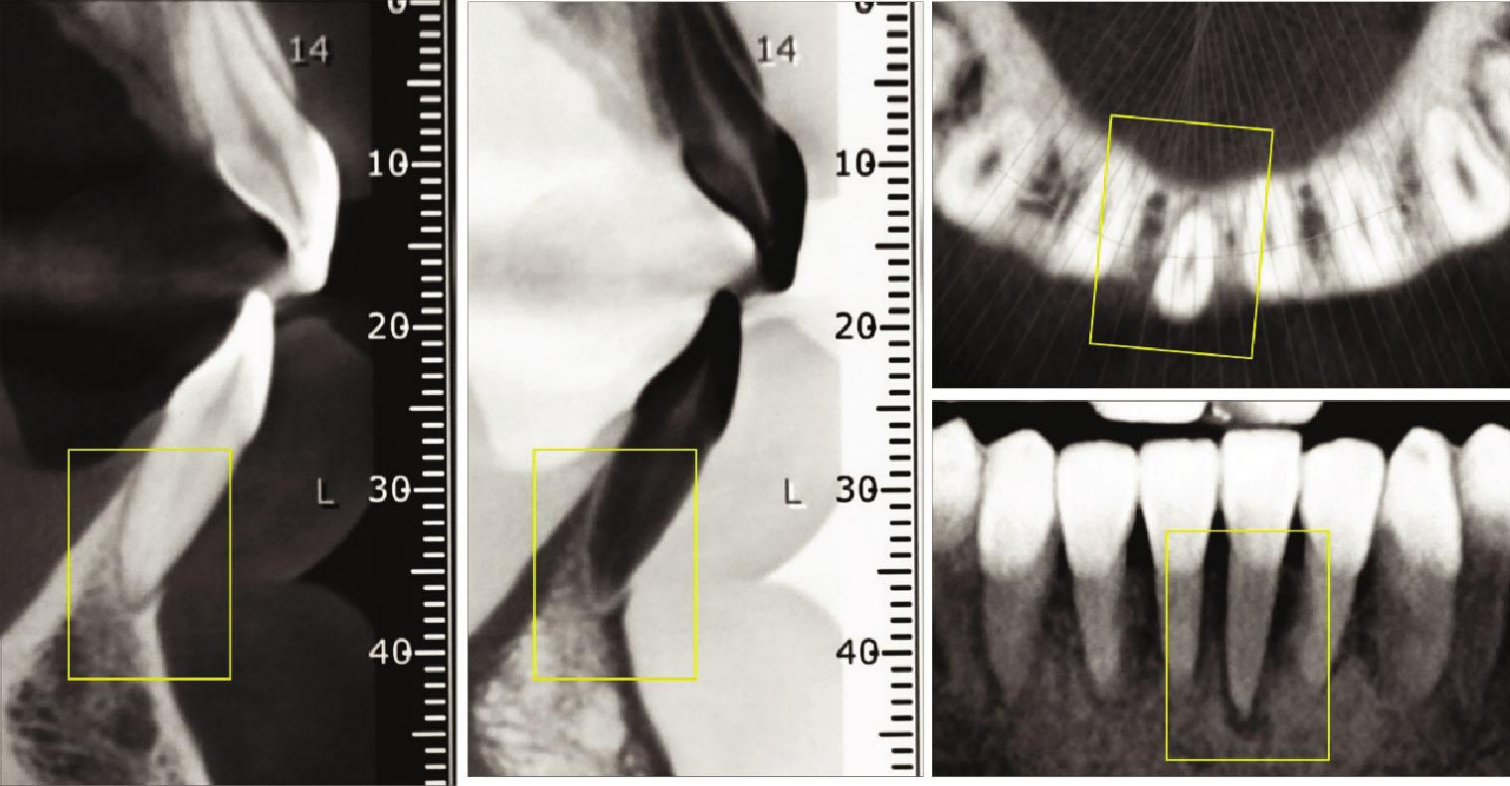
Initial CBCT of the left mandibular central incisor. Sagittal, coronal, and axial views.
